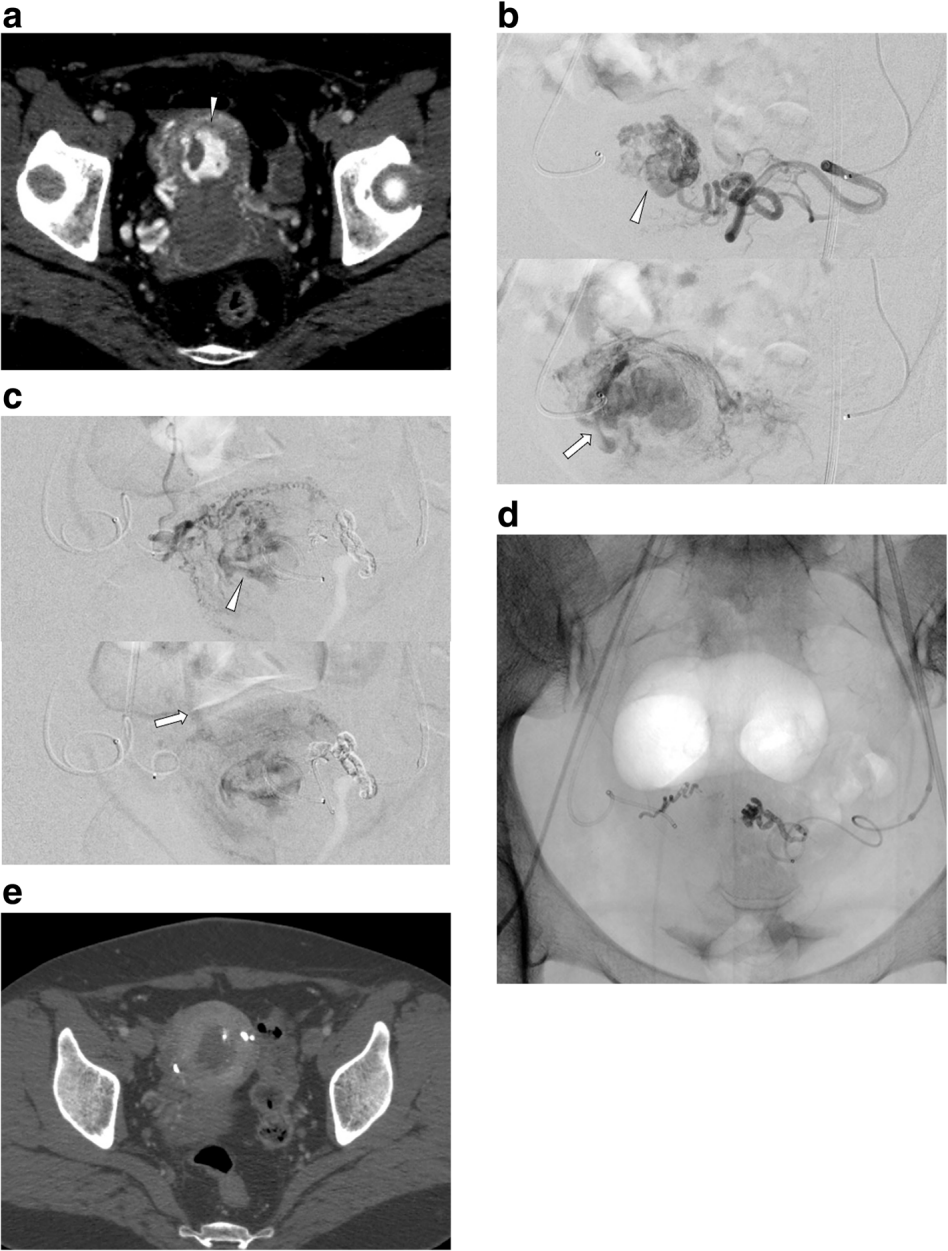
A 42-year-old woman with vaginal bleeding caused by retained placenta. a. Arterial phase CE-CT showed intrauterine enhancing lesion (arrowhead). b, c. Bilateral uterine arteriogram showed dilated vascular channel (arrowhead) in the arterial phase. Venous drainage (arrow) was depicted in the capillary phase through the vascular channel. d. Bilateral uterine arteries were embolized using 25% NBCA diluted with iodized oil. After the TAE procedure, expectant management was chosen and no re-bleeding was observed. e. At 1 month after the TAE procedure, the intrauterine enhancing lesion disappeared on CE-CT
